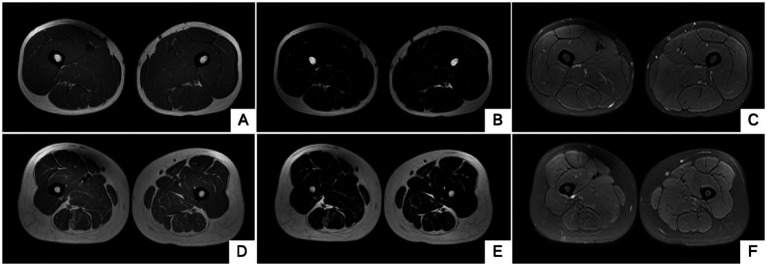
Muscle MRI of two probands’ thighs. No anomalies found. MRI of both thighs were taken at the level that is approximately 25 cm above the knee. (A–C) Proband 1 III-5. (D–F) Proband 2 II-3. A/D, B/E and C/F are T1WI, T2 water suppression and T2 fat suppression, respectively.
